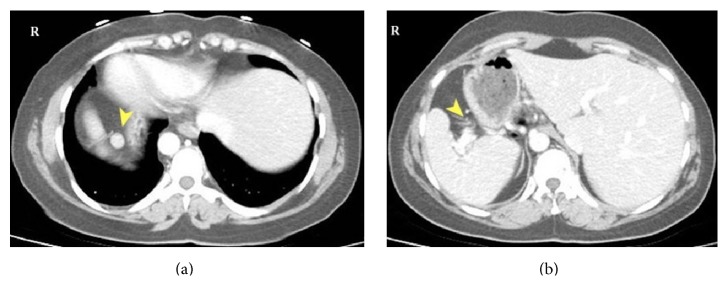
Abdomen CT; mirror view of intra-abdominal organs due to situs inversus, accessory spleen (arrow head (a)), and no pancreatic tissue in splenic hilum (arrow head (b)).
